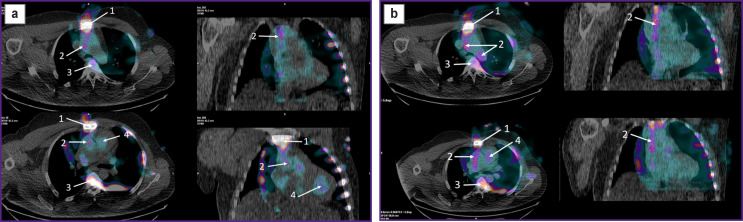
SPECT/CT images of the chest (axial slices — on the left; frontal slices — on the right) of patient P., performed 3 (a) and 6 h (b) after the administration of ^99m^Tc-pyrophosphate The arrows point to the mediastinal structures, which produce significant artifacts and do not allow clearly identifying the pathological accumulation of RP in the ascending and descending aorta walls. *1* — sternum, *2* — radioactive blood pool in the ascending aorta, *3* — spine, *4* — radioactive blood pool in the left ventricle
